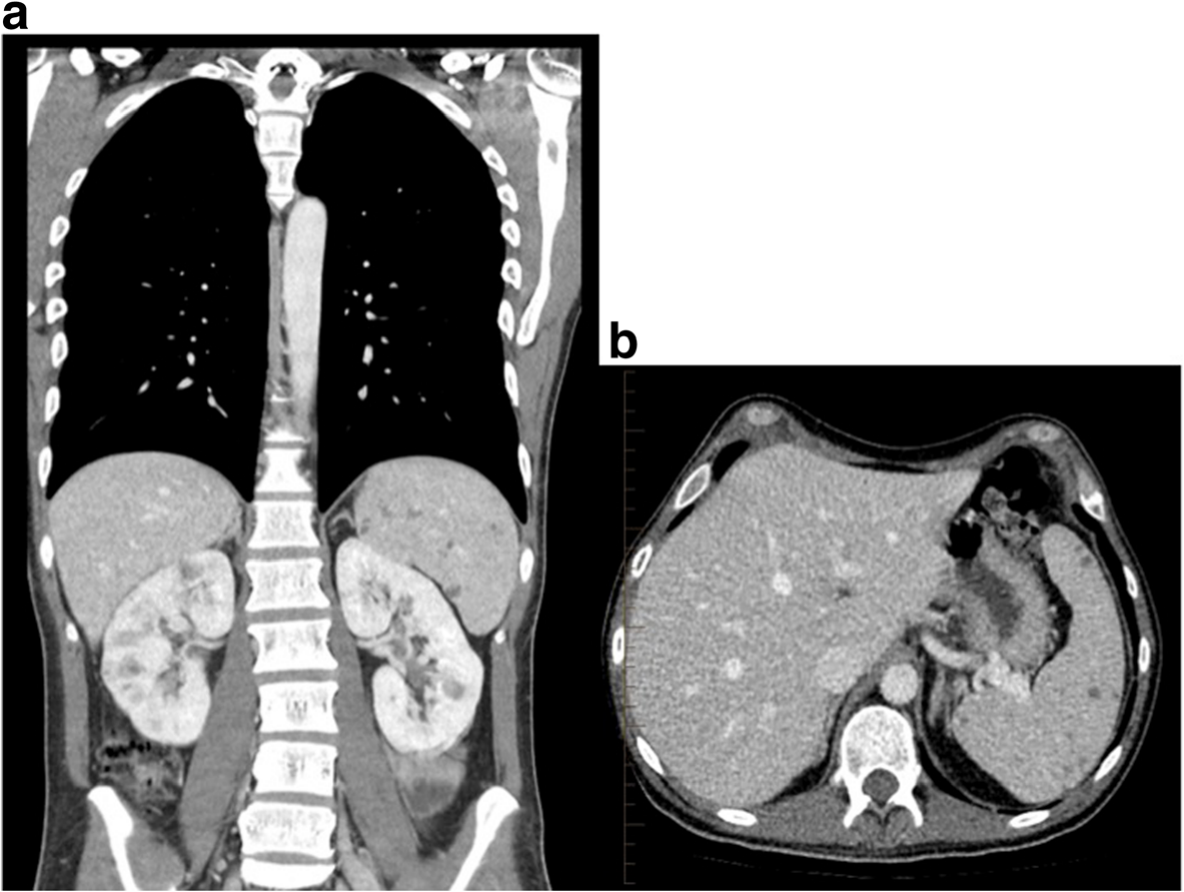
Computed Tomography (CT) of the chest (a) and abdomen (b) demonstrated left axillary adenopathy, innumerable small hypodensitities in the spleen and multiple lesions in the kidneys
